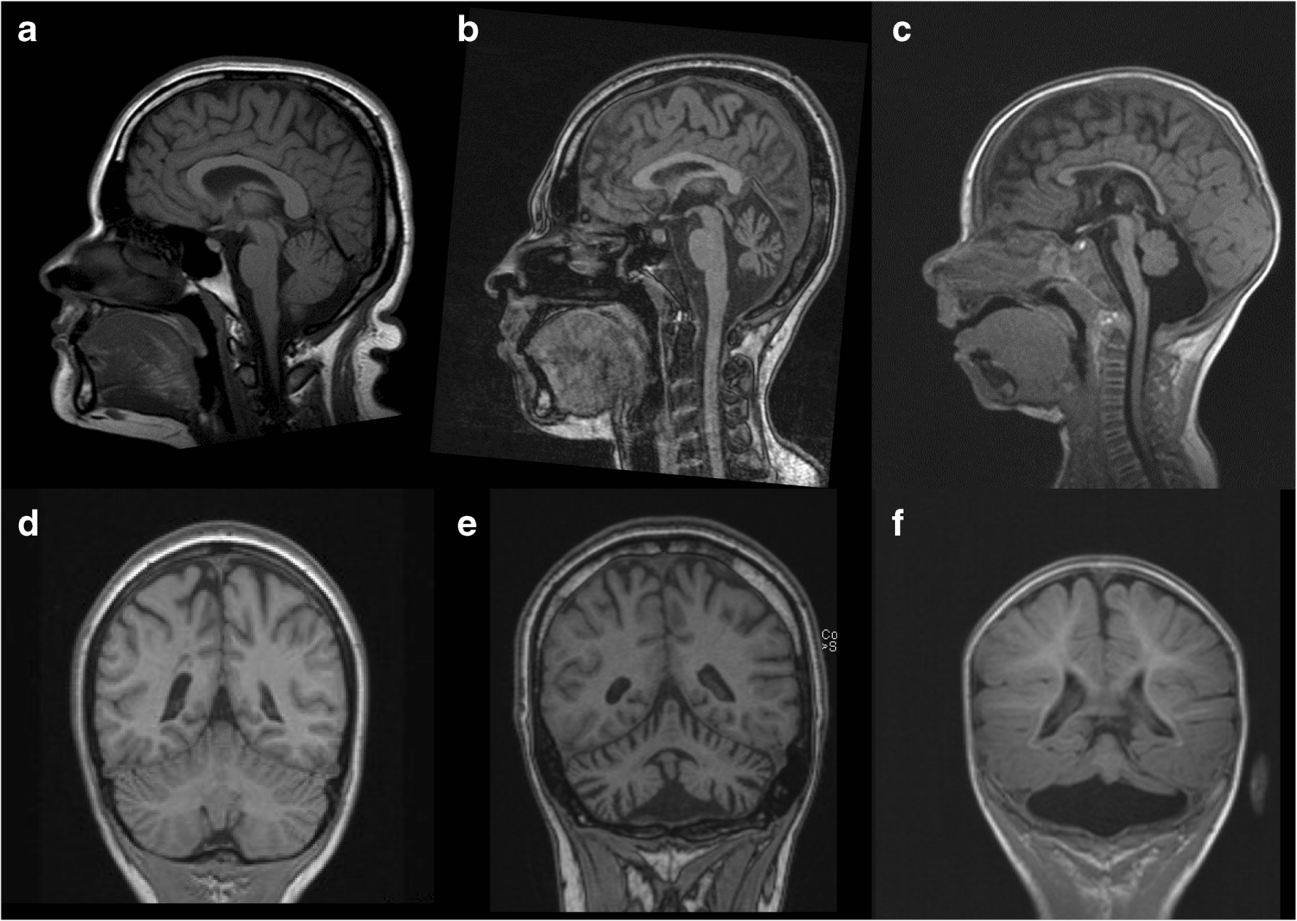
Brain MRI showing cerebellar atrophy in our patient, compared to a control and a PCH2A patient. (a) T1-weighted midsagittal image of a control. (b) T1-weighted midsagittal image of our patient, aged 23. Enlarged extracerebellar liquor spaces indicate atrophy of cerebellar vermis. The pons is normal. (c) Midsaggital MPR-image of a PCH2A patient at 6 months of age shows severe flattening of the ventral pons and hypoplasia of the cerebellar vermis. (d) MPR coronal image of control. (e) MPR coronal image or our patient shows atrophy of cerebellar hemispheres. (f) MPR coronal image of PCH2A patient indicates severe cerebellar hypoplasia with relative sparing of the vermis, resulting in a typical dragonfly pattern
